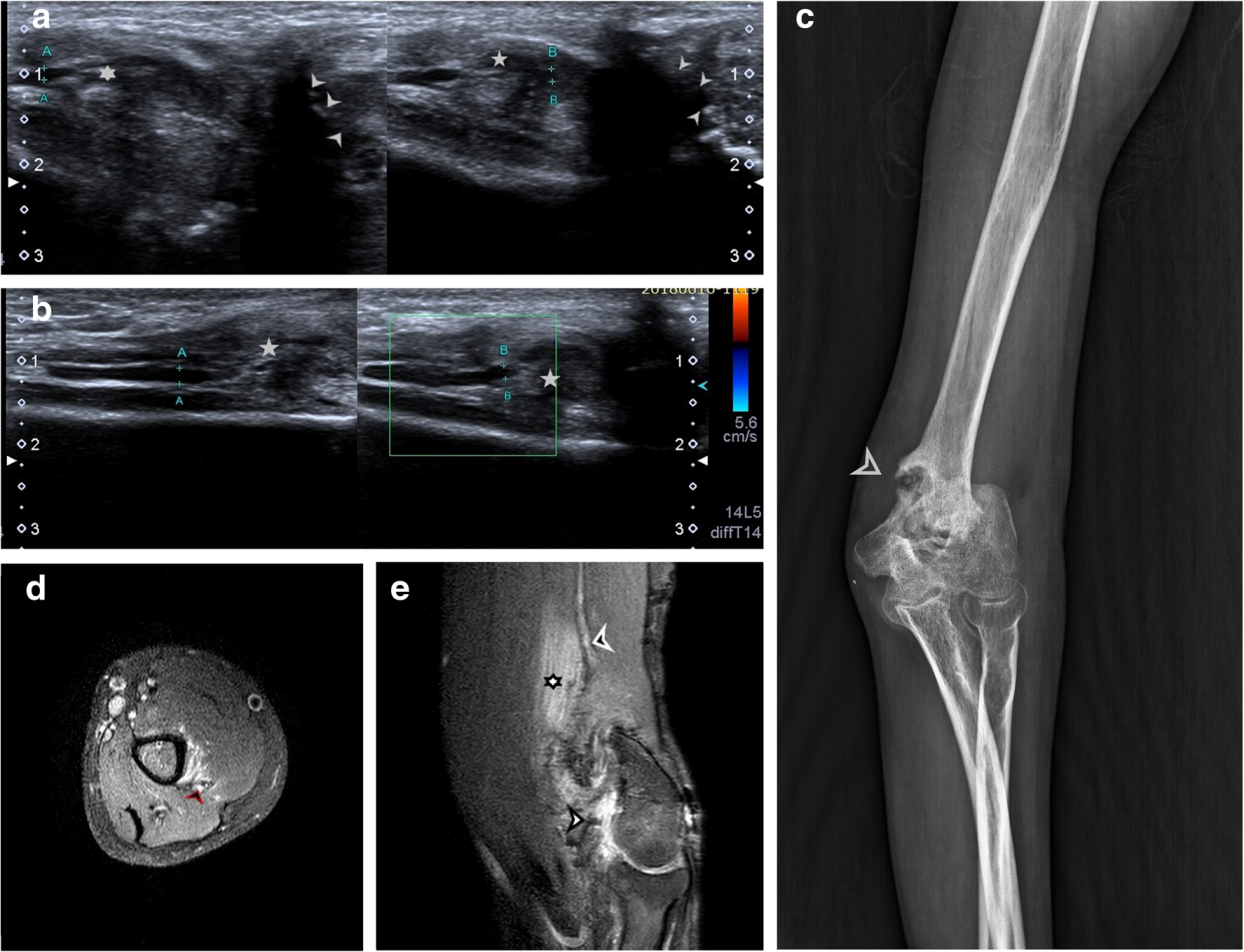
a Hypoechoic radial nerve (point A and B) is seen entrapped within mixed echogenic chronic inflammatory soft tissue (5 point and 6 point star). Malunited fracture of humerus can be seen adjacent to it (arrowheads). b Long axis view of hypoechoic radial nerve (point A and B) seen entering into the chronic inflammatory tissue. There was no evidence of increased vascularity of the nerve. c Plain radiograph showing malunited, displaced fragment of supracondylar fracture of humerus. d Axial PD sequence showing hyperintense radial nerve (black arrowhead) with surrounding hyperintense muscle oedema. e Sagittal PD sequence showing heterogeneously hyperintense area of chronic inflammation in region of malunited supracondylar fracture of humerus (white arrowhead). The radial nerve is enlarged and hyperintense (black arrowhead) and is not visualised within the area of chronic inflammation and surrounding muscle oedema (6 point star) due to similar signal intensities
